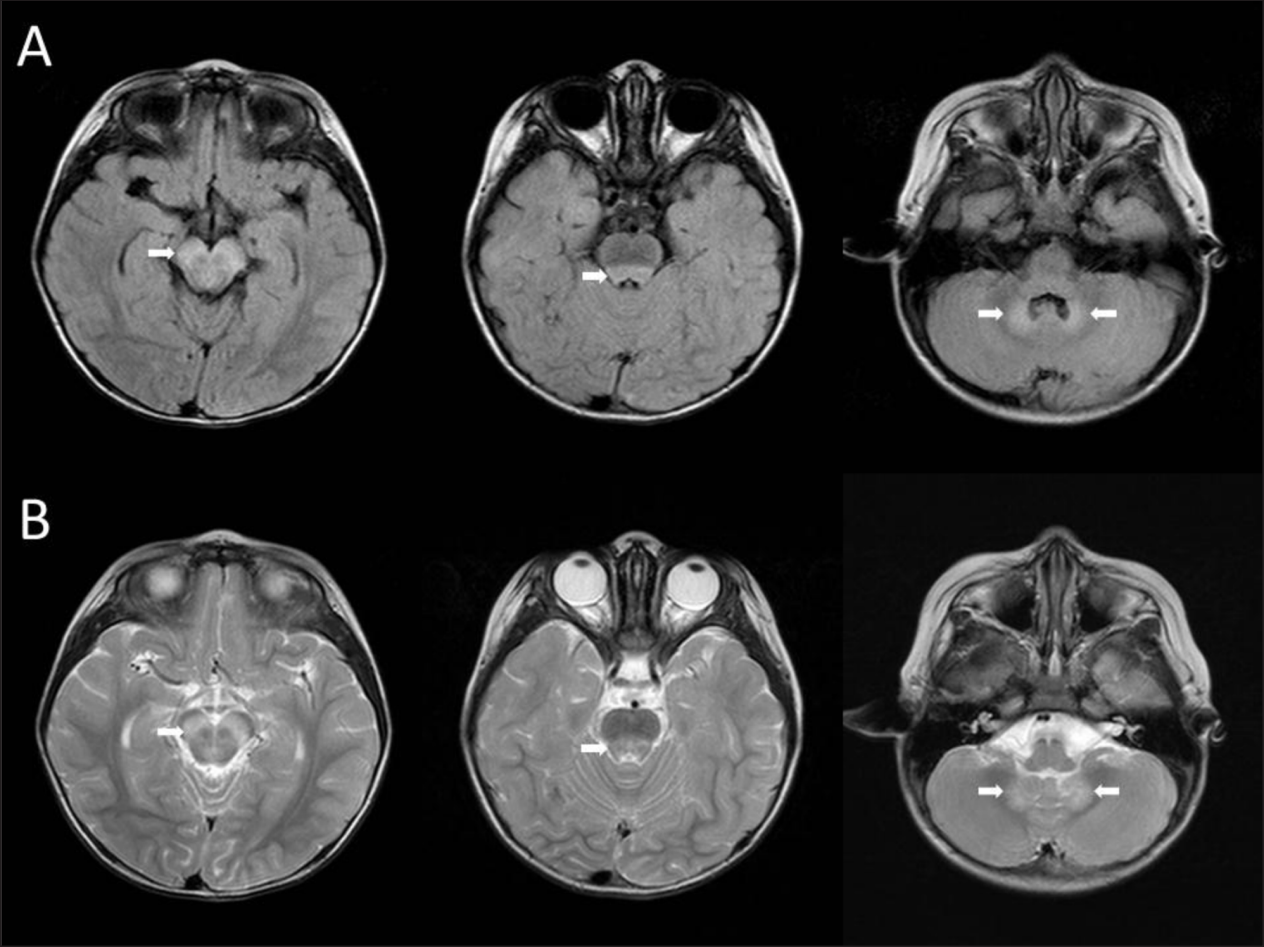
FLAIR (A) and T2WI (B) demonstrate hyperintense lesions in the midbrain, dorsal pons, and cerebellar dentate nuclei (white arrows).
